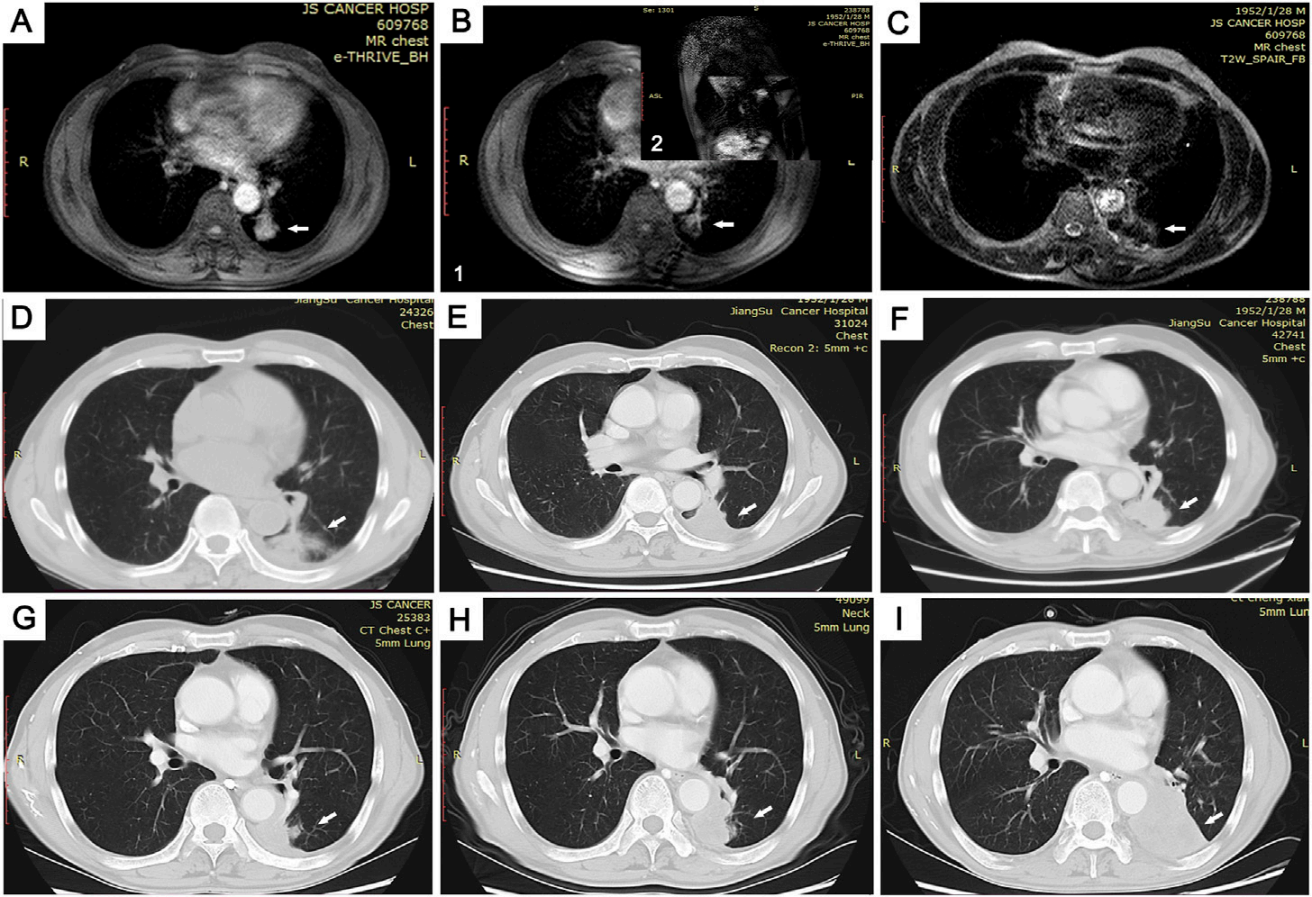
Images of the MRI-guided MWA procedure and follow-up in 69-year-old man with pulmonary adenocarcinoma in inferior lobe of left lung. (A) Tumor lesion seen on MRI immediately prior to MWA. (B) Combined with axial (1) and sagittal (2) MRI scans, the ablation needle reached the tumor site (C,D). By comparing T2WI MRI scan images (C) and lung window CT images (D), we found MRI to be more accurate in the assessment of microwave-ablation boundaries. (E-I) Follow-up CT scans at 3 (E), 6 (F), 12 (G), and 20 (H) months showed no change in the GGO size. At the 26-month follow-up (I), the tumor size was increased.
